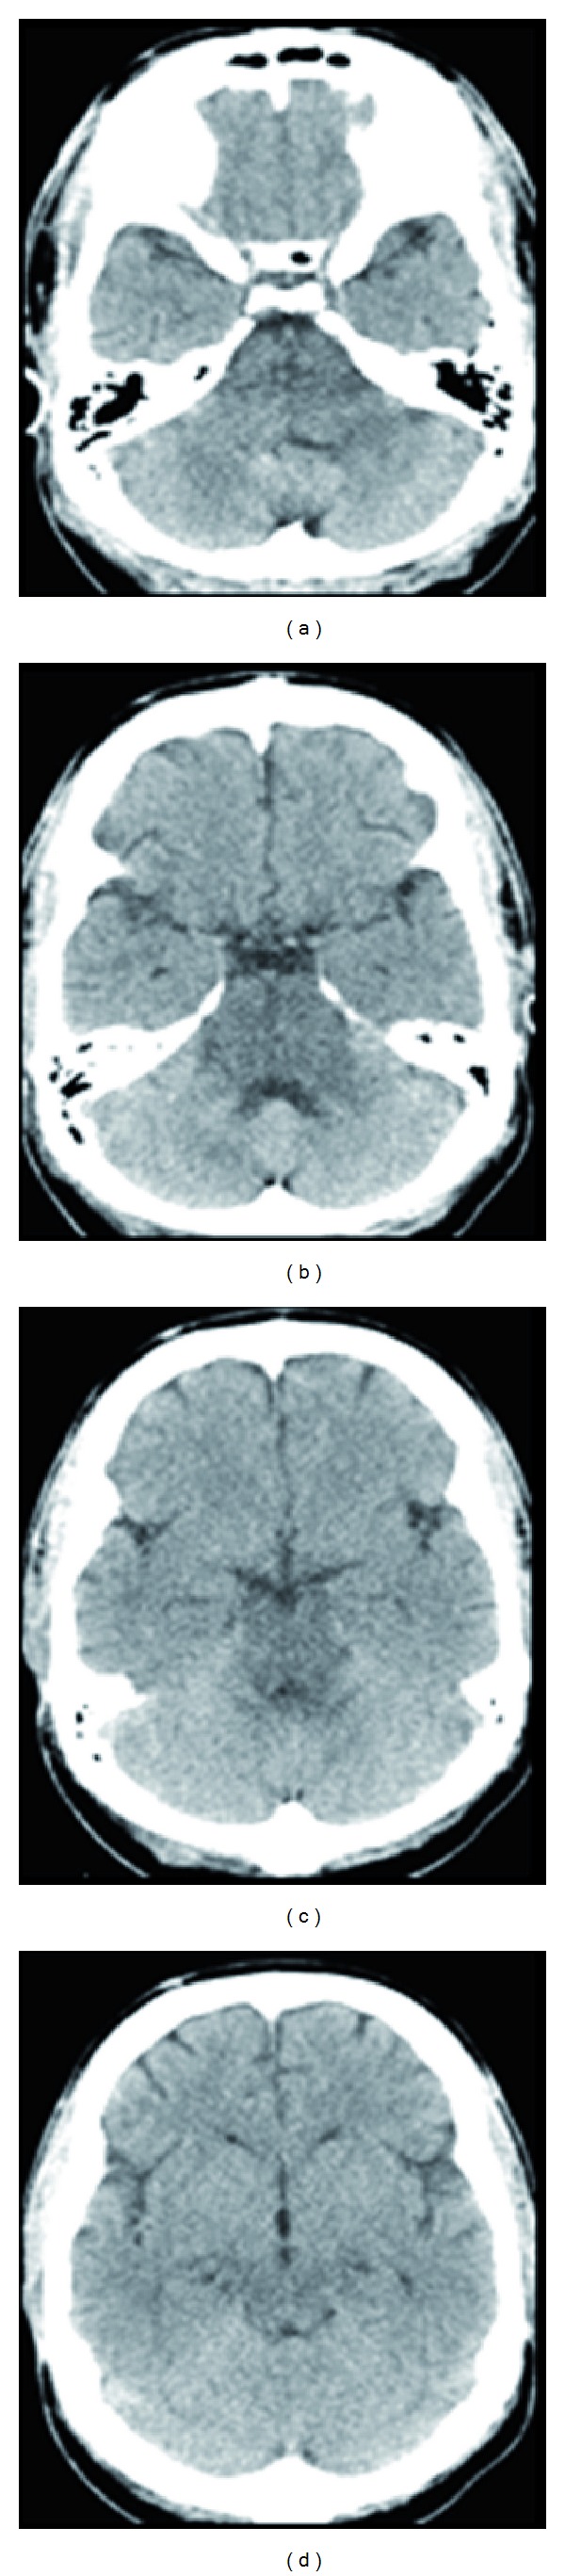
Noncontrast brain CT scan showing isolated hypodensity of the whole Pons ((a) and (b)) and midbrain ((c) and (d)) with narrowed prepontine, cerebellopontine, and ambient cisterns.
